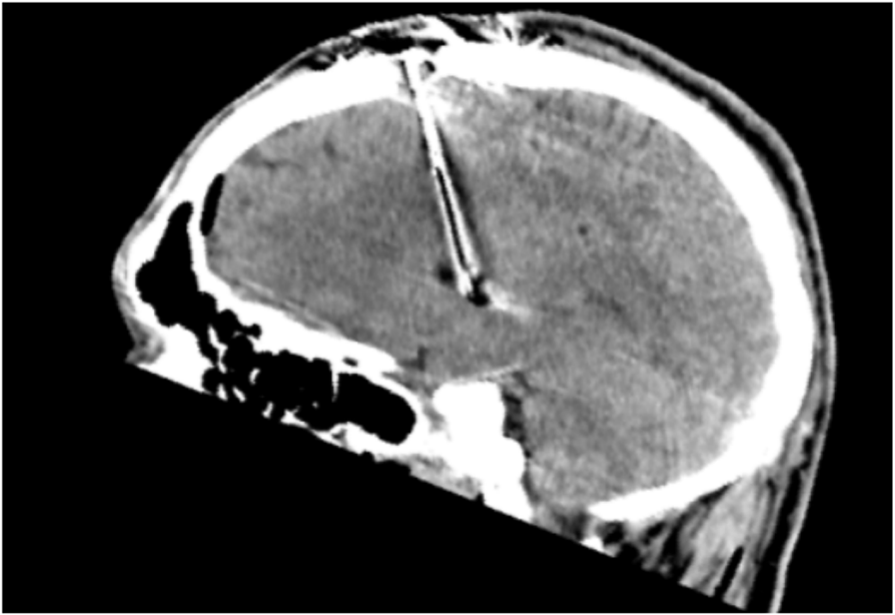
CT scan of brain (immediately after surgery, sagittal view). Without bleeding along the left electrode. The image quality is partially affected by artifacts from the electrode (electrode – black color; artifact around the electrode - white color)
